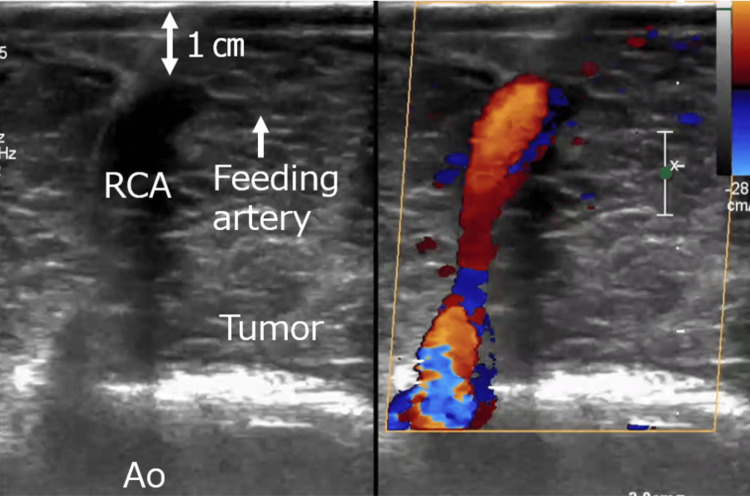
Intraoperative periepicardial echocardiography. The course of the coronary artery within the tumor was visualized using periepicardial echocardiography. RCA: right coronary artery
